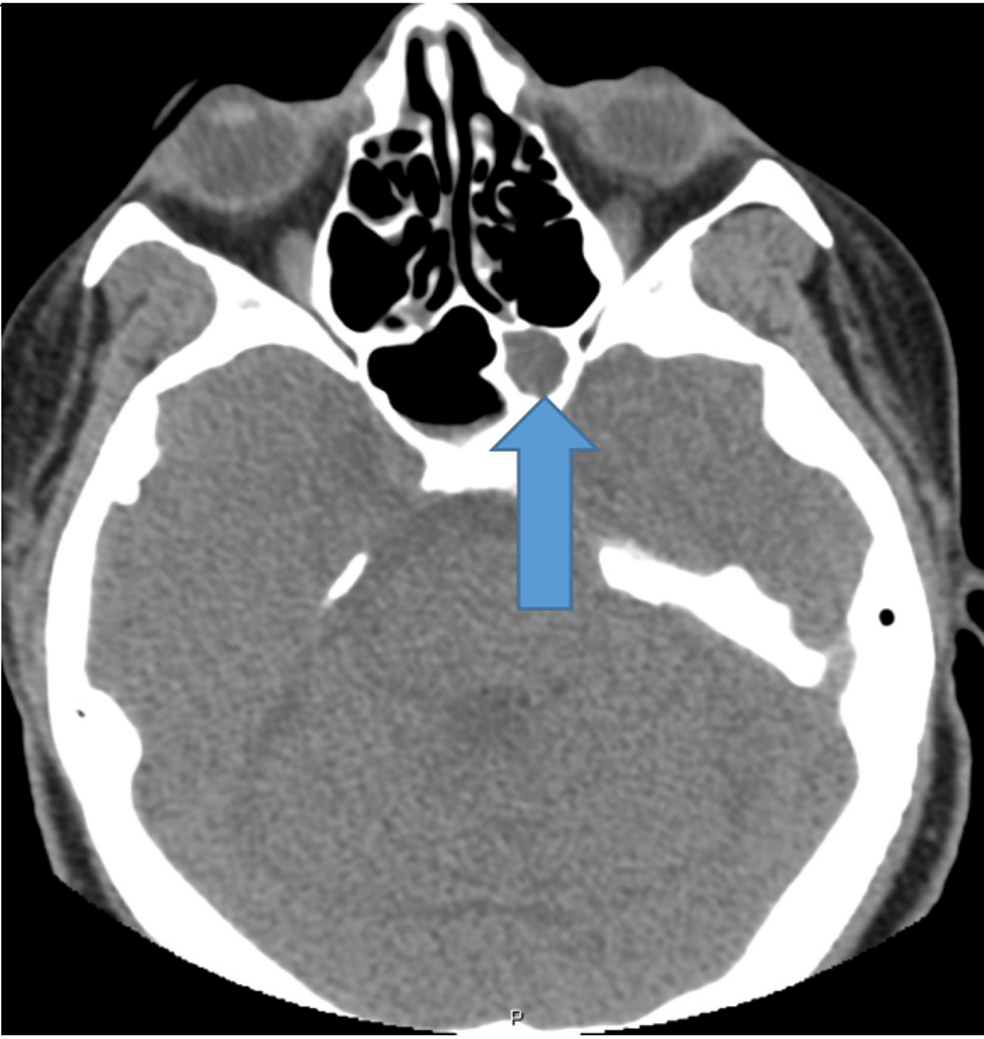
CT maxillofacial with blue arrow pointing to opacified left sphenoid sinus
